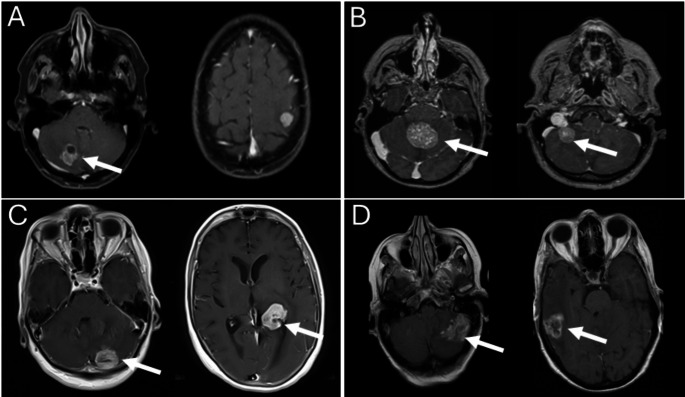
Preoperative MRI (T1 weighted, with contrast agent) of BC patients with multiple BM. A: Resection of 1 cerebellar BM, B: resection of 2 cerebellar BM, C: one stage operation with two craniotomies for resection the cerebellar and the ventricular infiltrated BM, D: two-staged operation, first with the resection of a cerebellar BM, followed by a second surgery in 6 months for the resection of the temporal BM. The white arrow shows the metastasis that was/were removed. Abbreviations: BC: breast cancer, BM: brain metastases, MRI: Magnetic Resonance Imaging
